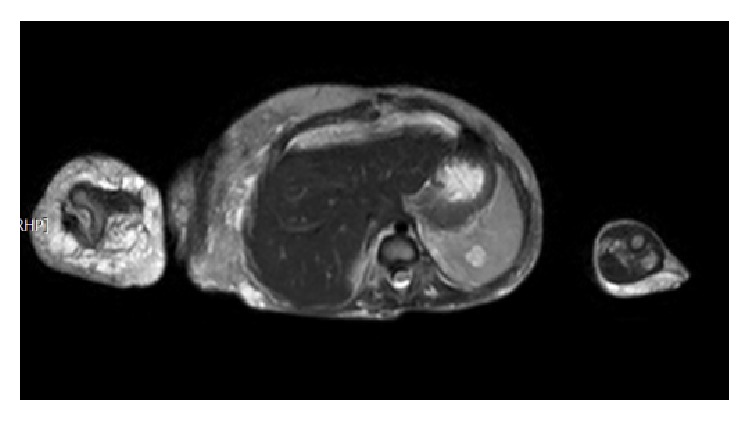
MRI of the abdomen showed the hemangioma in the spleen.
